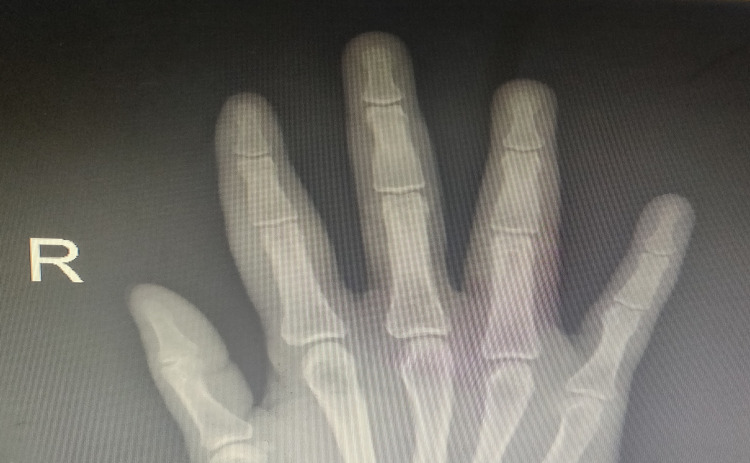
Hand X-Ray depicting mild edema of the soft tissue of the right index and ring fingers
